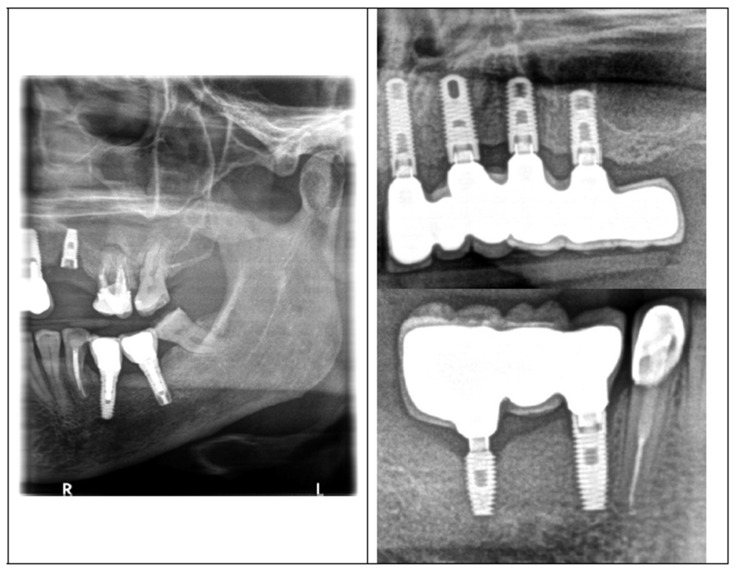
X-ray exemplary imaging of the screw failures: left X-ray, loose/broken screw in the upper left premolar CsNo8; upper-right X-ray, broken screws in implants 21 and 23 and loosened screws in implants 24 and 25 CsNo2; lower-right X-ray, broken hex in implant 46, both screws in 44 and 46 were loose in CSNo7.
